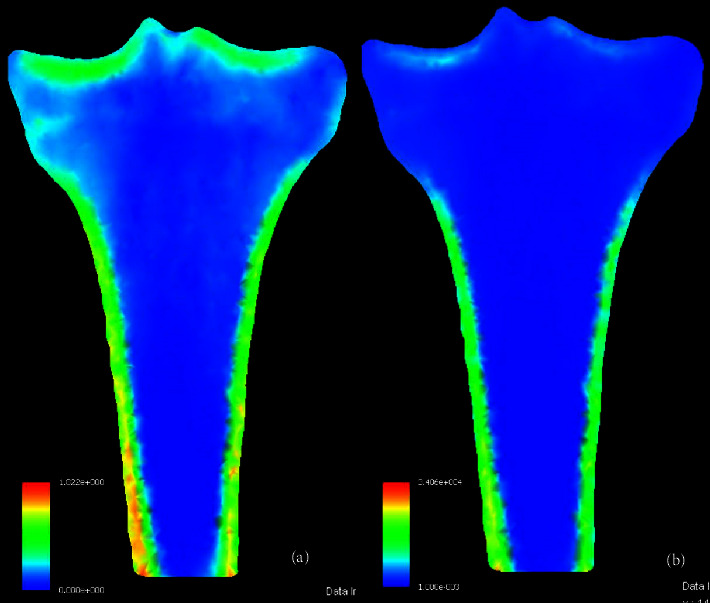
(a) Density (mg/mm^3^) and (b) Young's modulus (MPa) of the tibia model after conversion of CT values through Keyak's formula.
